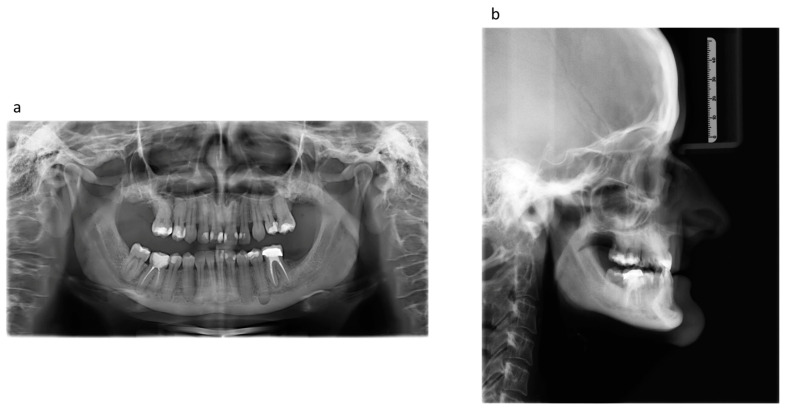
(a) Orthopantomography before any orthodontic and surgical intervention: note the typical hypercementosis with apical radiopaque halos and the dental roots superimposing the mandibular canal. (b) The lateral cephalogram before any orthodontic and surgical intervention: skeletal class III according to ANB (−2.2°) and WITS (−6.6 mm) with an orthognathic maxilla and a manifest prognathous mandible.
